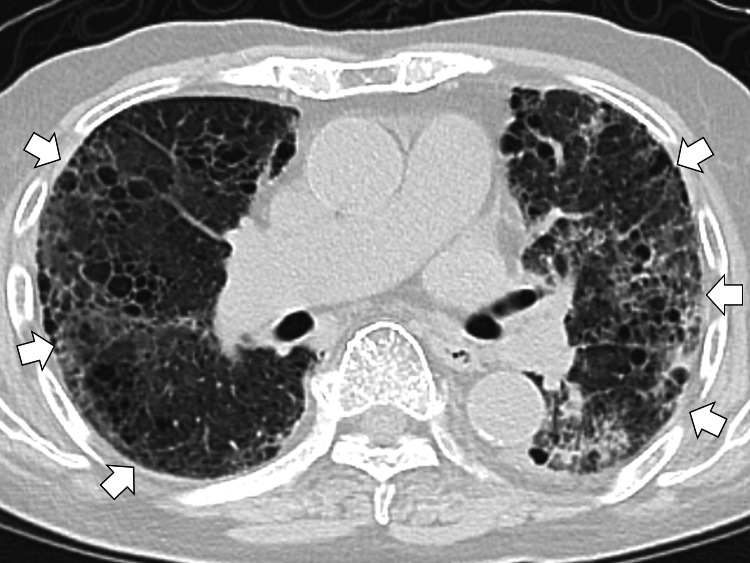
The computed tomography of the chest showing bilateral diffuse interstitial filtration (white arrows)
